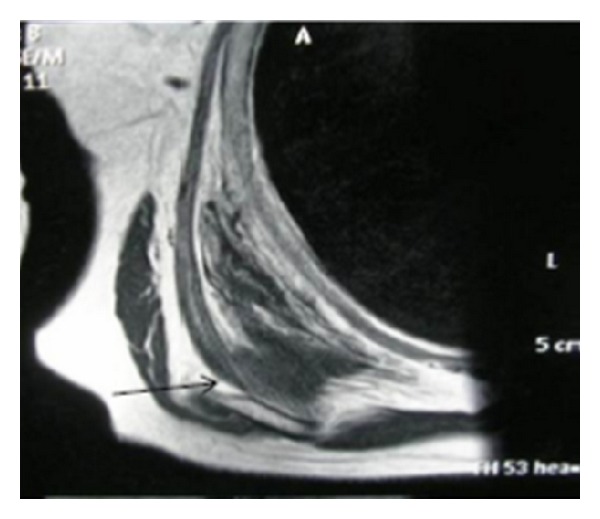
Axial MRI image indicating a hypointense rather round mass with signal intensity almost equal to surrounding muscles.
